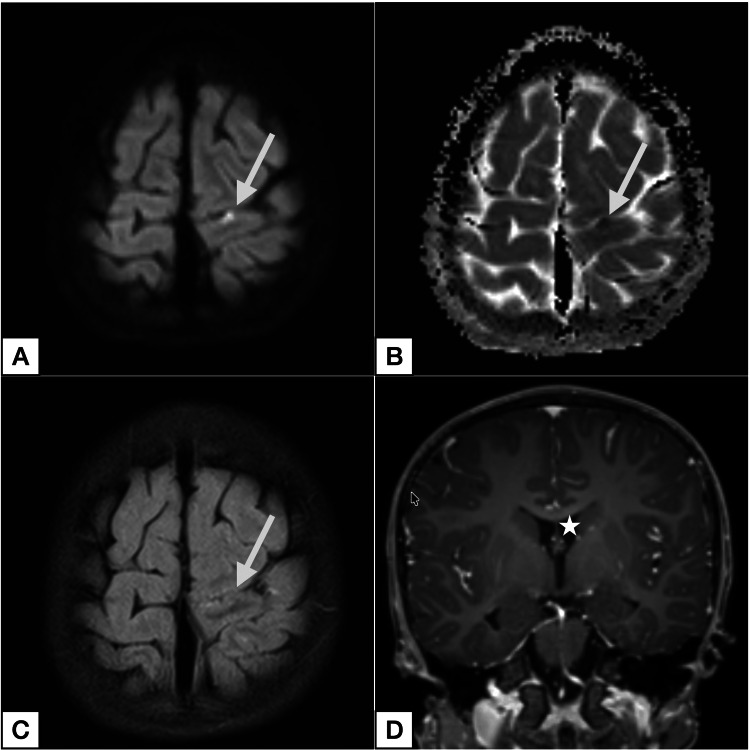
Magnetic resonance imaging at initial ischemic presentation (nine months prior to aneurysmal subarachnoid hemorrhage) (A) Axial diffusion-weighted imaging demonstrating focal cortical diffusion restriction in the left precentral gyrus (arrow). (B) Corresponding apparent diffusion coefficient map (arrow) confirming true restriction with matching hypointensity. (C) Axial fluid-attenuated inversion recovery sequence without clear lesion demarcation (arrow), consistent with acute ischemia. (D) Coronal contrast-enhanced T1-weighted imaging demonstrating normal ventricular size (asterisk).
